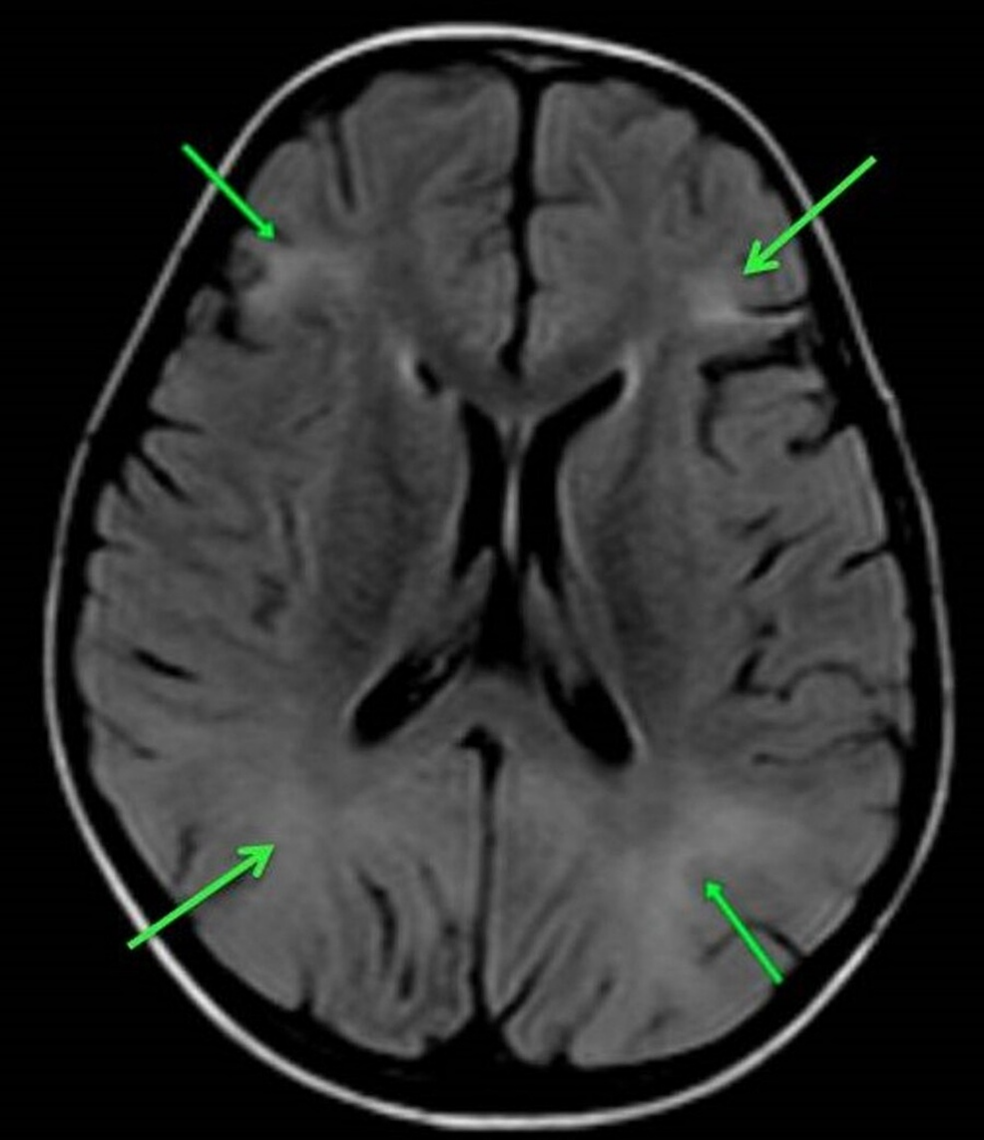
Axial fluid-attenuated inversion recovery (FLAIR) image Confluent hyperintensities involving the subcortical and deep white matter along bilateral frontoparietal regions (green arrows).
